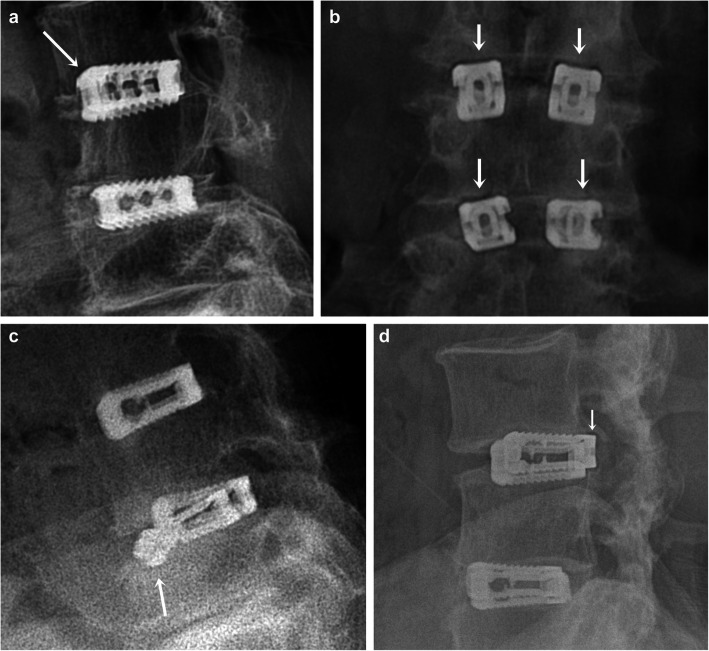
Implant failures after follow-up. a Lateral radiograph showing subsidence. b Anterior-posterior radiograph showing pseudoarthrosis. c Lateral radiograph showing cage breakage. d Lateral radiograph showing retropulsion
